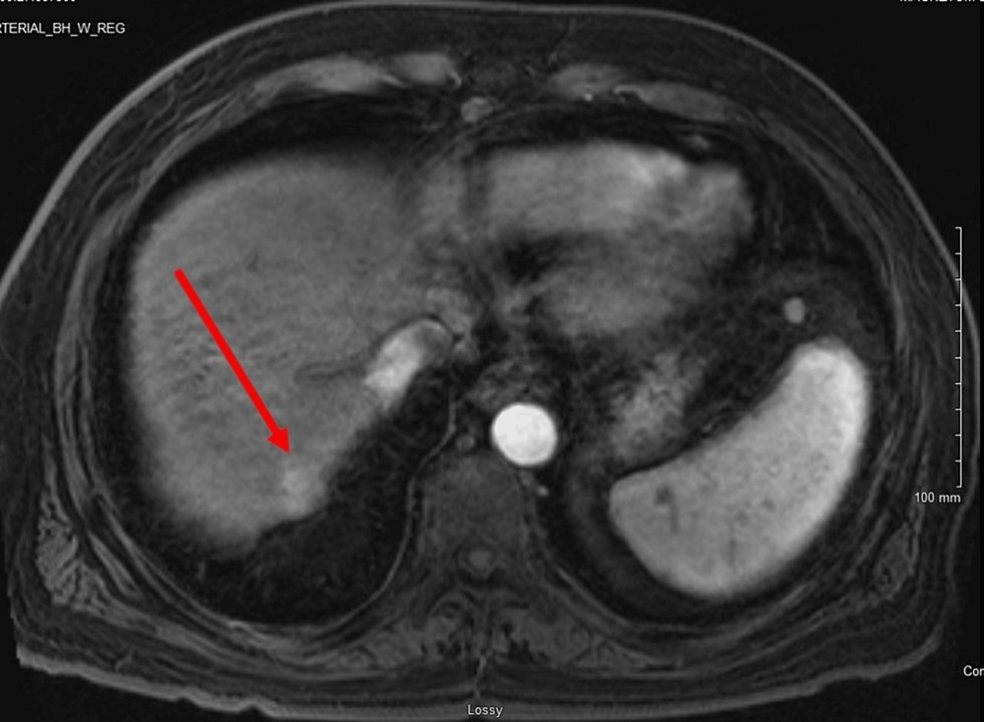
MRI of segment VII lesion MRI of the segment VII hepatocellular carcinoma lesion observed (arrow) with arterial phase enhancement.
